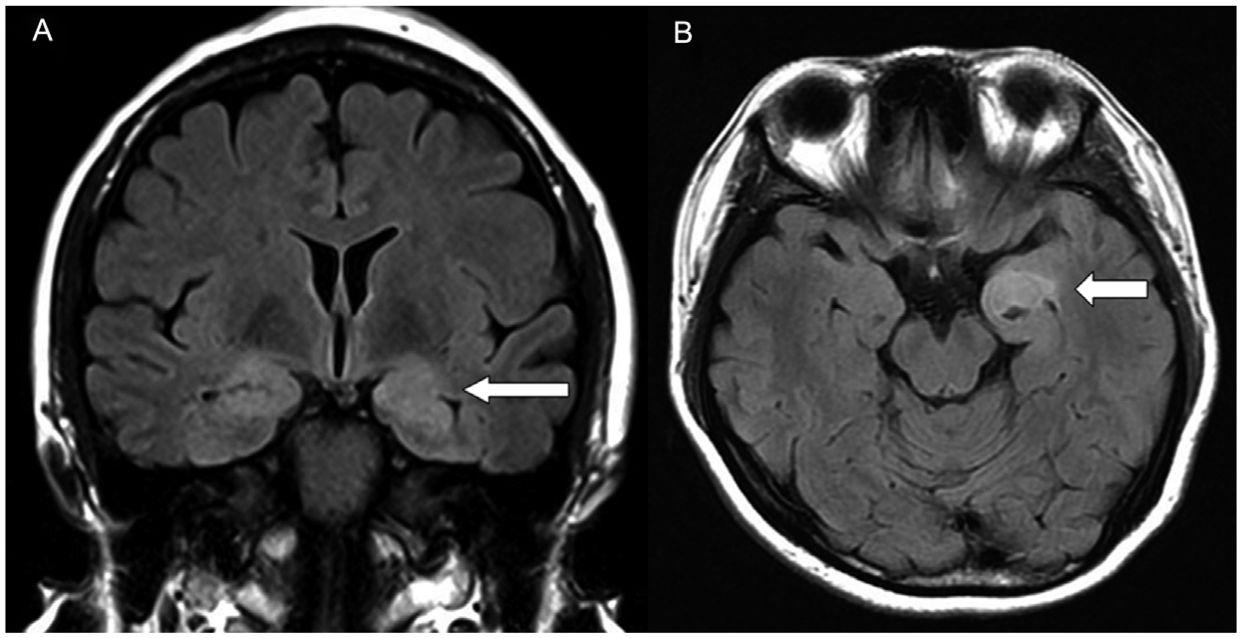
Limbic encephalitis-like manifestations on MRI images. (A) Coronal MRI image shows bilateral medial temporal and hippocampus hypersignal on FLAIR. (B) Axial MRI image shows left medial temporal and amygdala hypersignal on FLAIR.
